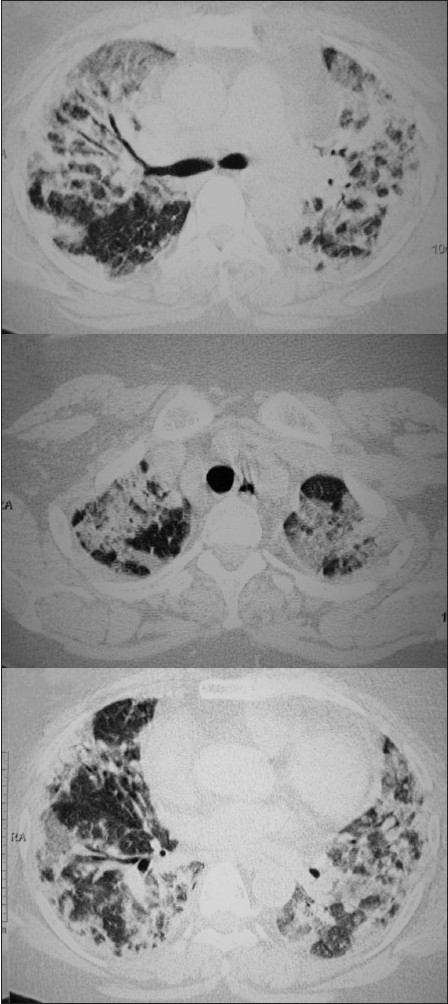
Lung windows of CT thorax showing bilateral multifocal consolidation and air bronchogram
